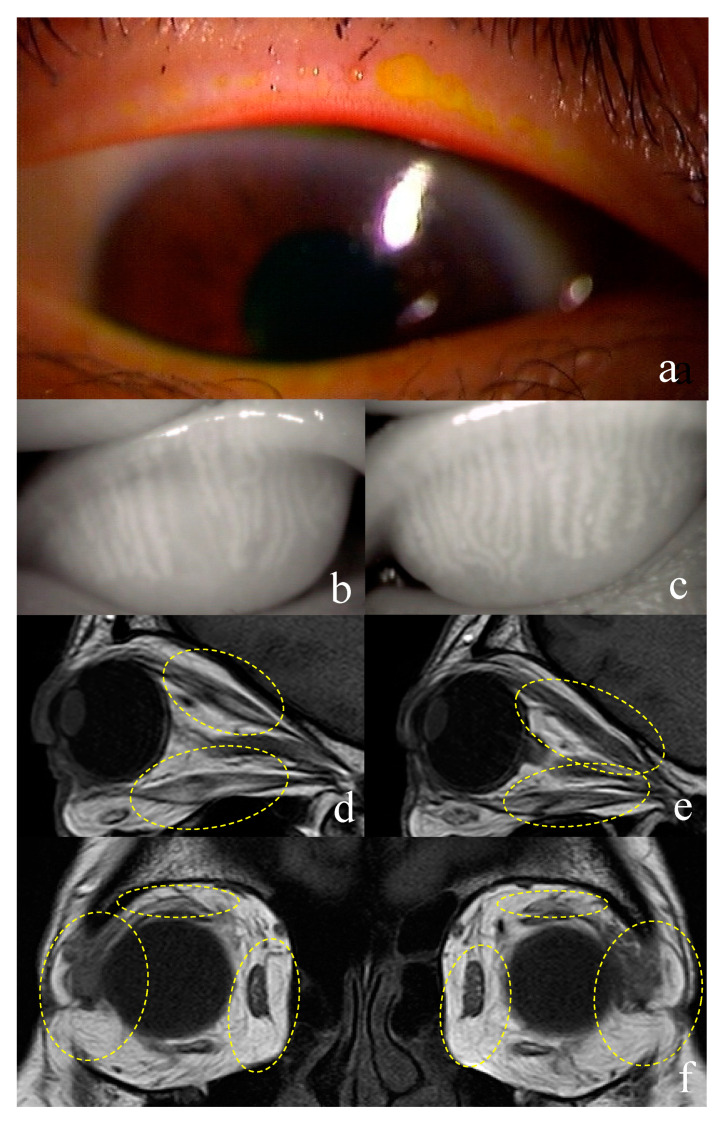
Representative case of a 56-year-old woman with Graves’ ophthalmopathy. The patient presented with lid-margin inflammation (a). The meibomian glands in the central region of the upper eyelid exhibit abnormal findings (b,c). Magnetic resonance imaging findings show (d,e) levator muscle and superior and inferior rectus muscle enlargement, as well as (f) medial rectus muscle enlargement and swelling of the lacrimal glands.
